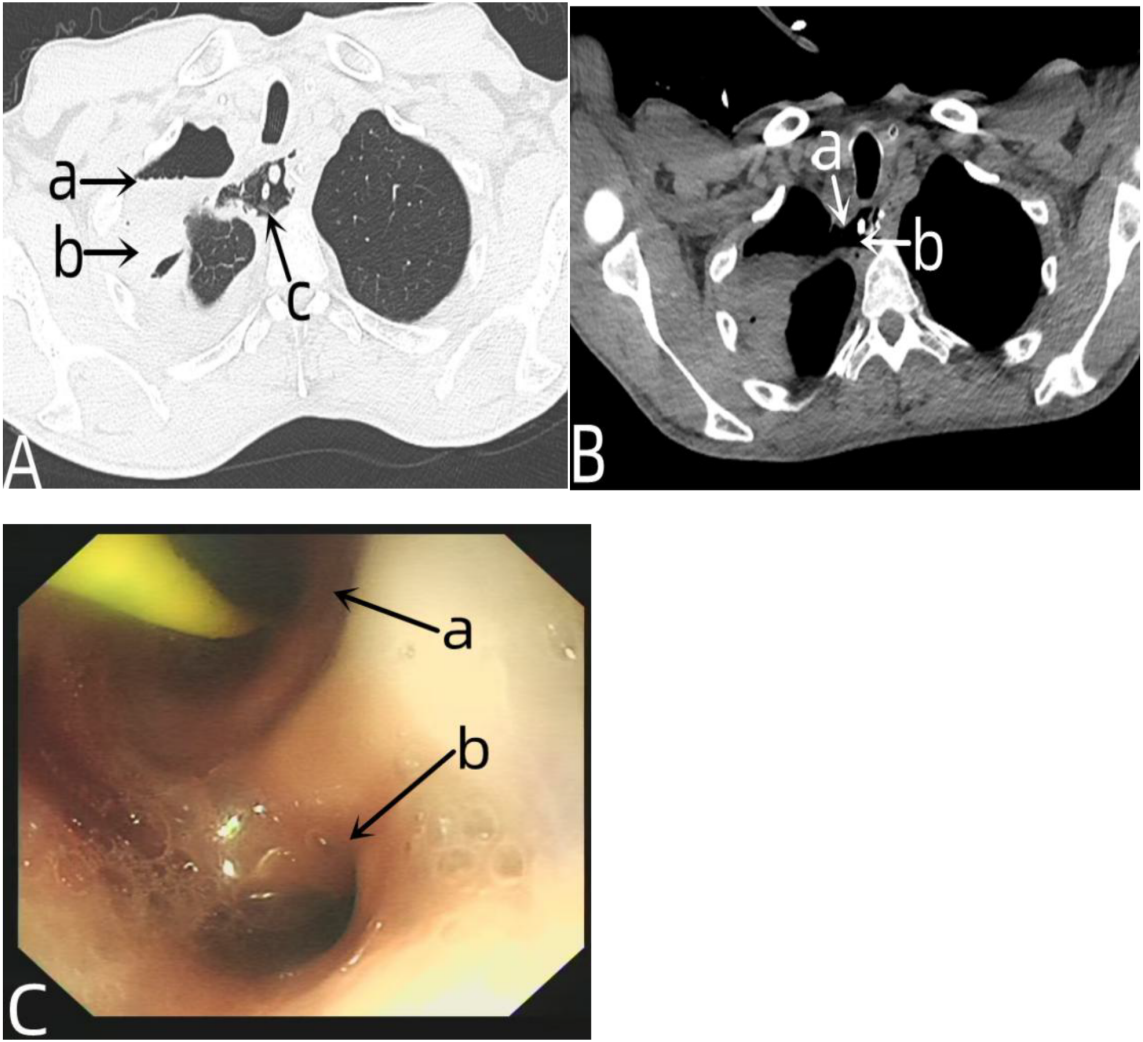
Chest CT and gastroscopy data of the patient with anastomotic leakage. In (A) the arrow a indicated the gas-liquid plane in the right thorax, the arrow b indicated the pleural effusion, and the arrow c indicated the tubular stomach in the thorax. In (B) the arrow a represented the fistula opening of the anastomotic site, and the arrow b represented the tubular stomach in the thoracic cavity. In (C) the arrow a and the arrow b respectively represented the lumen of the tubular stomach and the fistula of the anastomotic leakage observed under gastroscopy.
